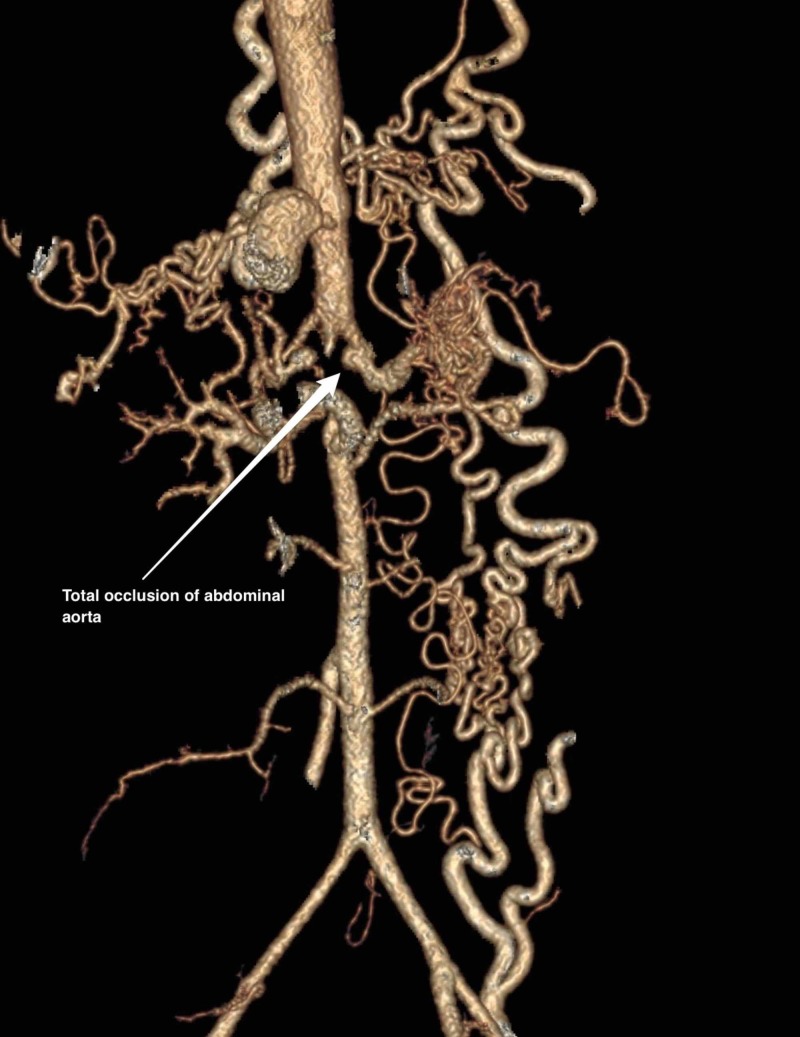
Computed tomography aortogram showing total occlusion of the abdominal aorta
